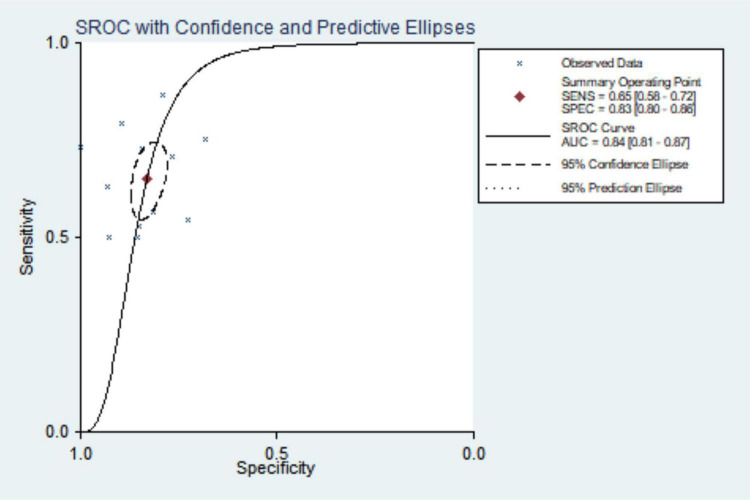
Summary receiver operating characteristic curve for CT diagnosis of pulmonary hypertension.
